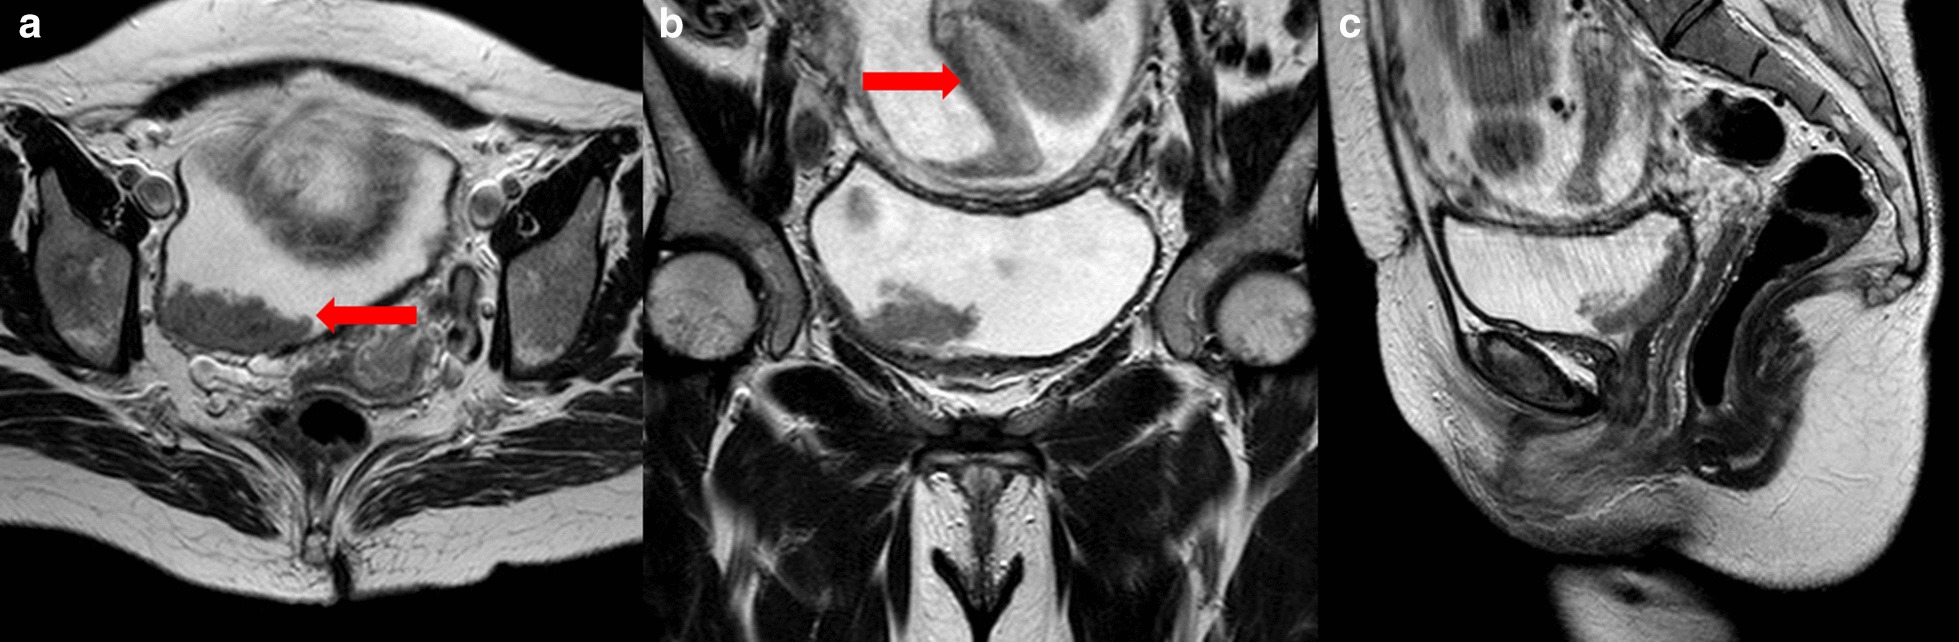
MRI without gadolinium at 23 weeks of gestation. a Axial view of the bladder tumor (arrow). b Coronal view of the bladder tumor and fetus (arrow). c Sagittal view
